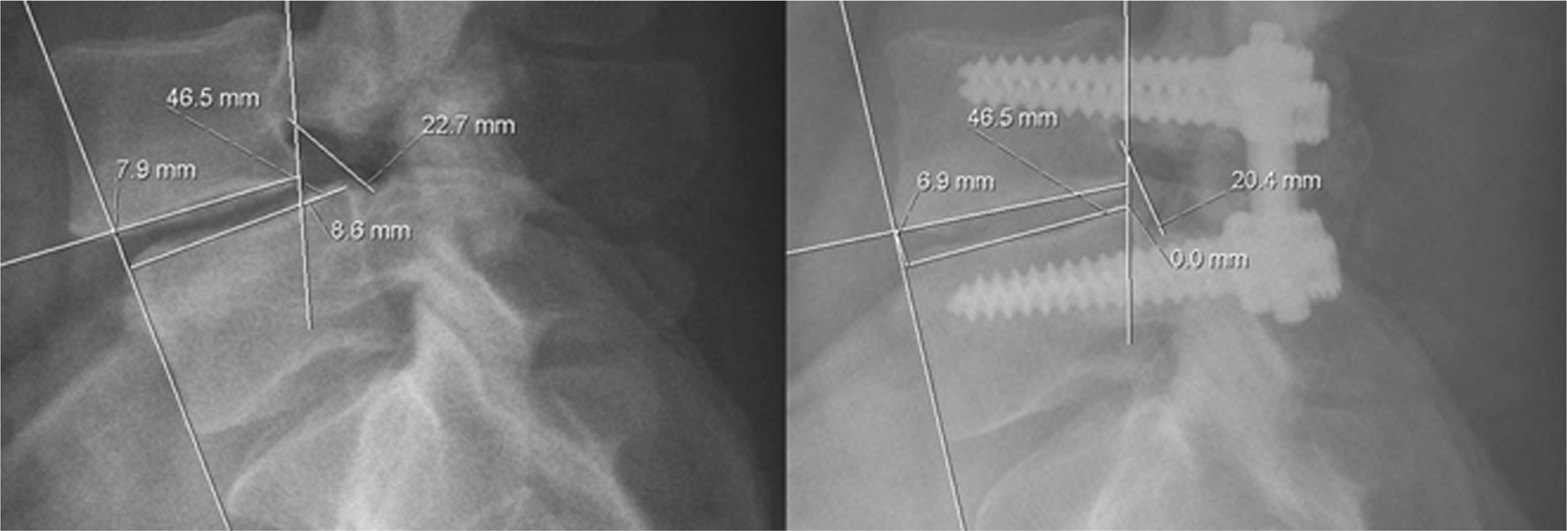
Typical example of radiographic parameter measurements preoperative and at 1 year follow-up. Preoperative listhesis of 8.6 mm was reduced to 0.0 mm, foraminal diameter was altered from 22.7 mm to 20.4 mm postoperatively. Anterior disc height decreased from 7.9 mm to 6.9 mm.
